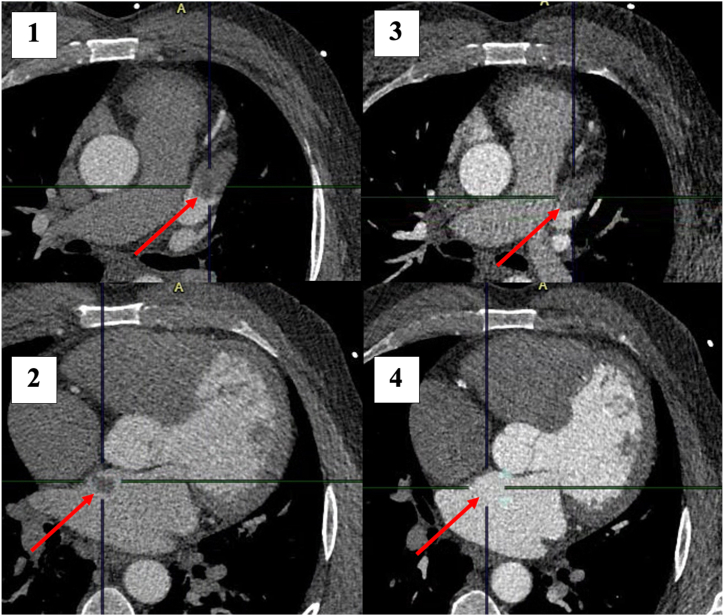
Patient with a left atrial appendage (1) and left atrial thrombus (2) on cardiac CT acquired during the initial stroke imaging protocol. Cardiac CT repeated after six days, showed a persisting left atrial appendage thrombus (3) while the left atrial thrombus was dissolved (4).
